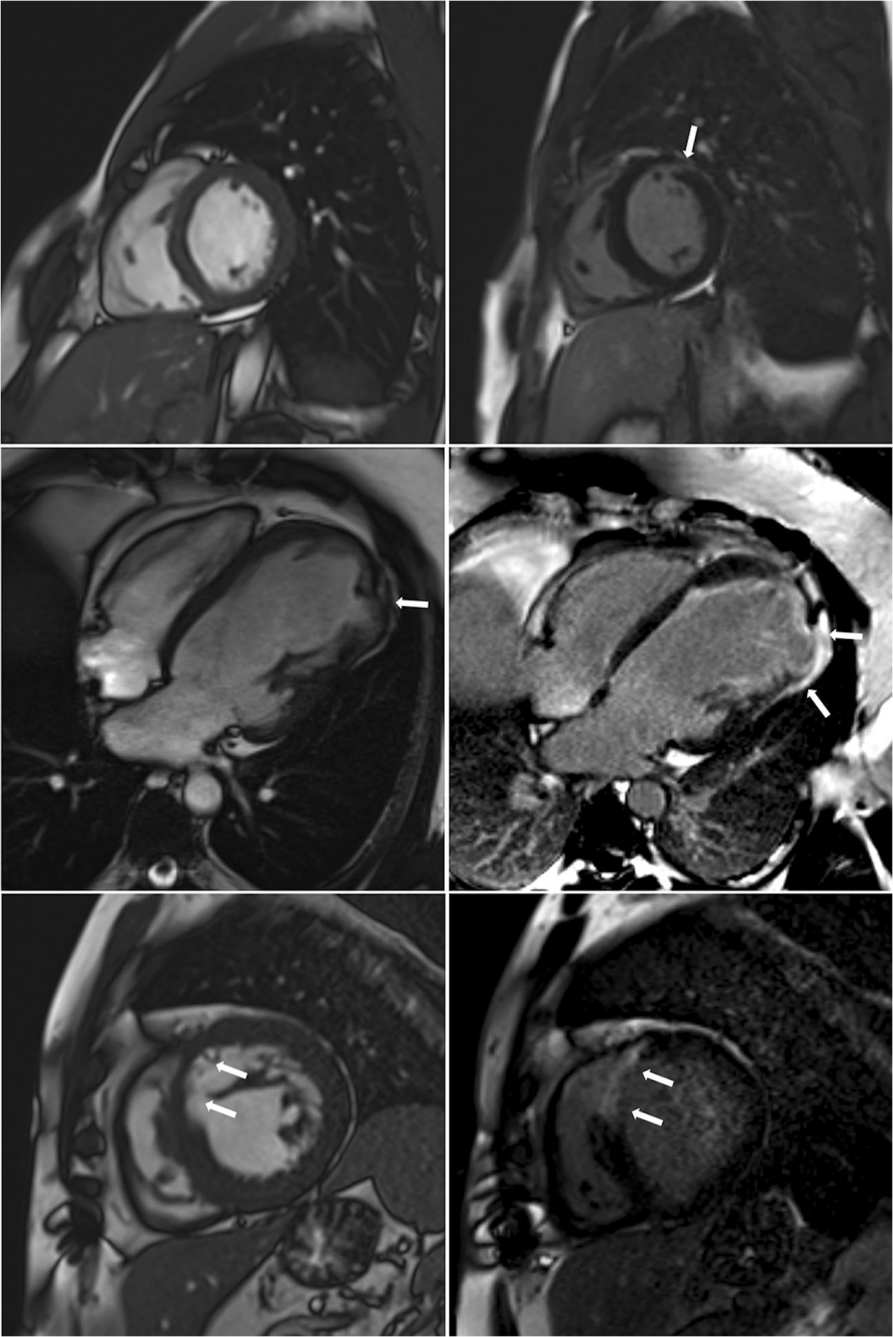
Diagnoses other than hypertrophic cardiomyopathy. Top row: left: only mild left ventricular hypertrophy with late gadolinium enhancement presenting a non-ischaemic pattern (right, arrow, status post-myocarditis). Middle row: left: aneurysm of the lateral wall apical segment (arrows) mimicking left ventricular hypertrophy in echocardiography; right: aneurysm of the lateral wall apical segment with transmural late gadolinium enhancement (arrows). Bottom row: left: “asymmetric” left ventricular hypertrophy due to prior myocardial infarction with subsequent wall thinning (arrows); right: transmural late gadolinium enhancement presenting an ischaemic pattern
